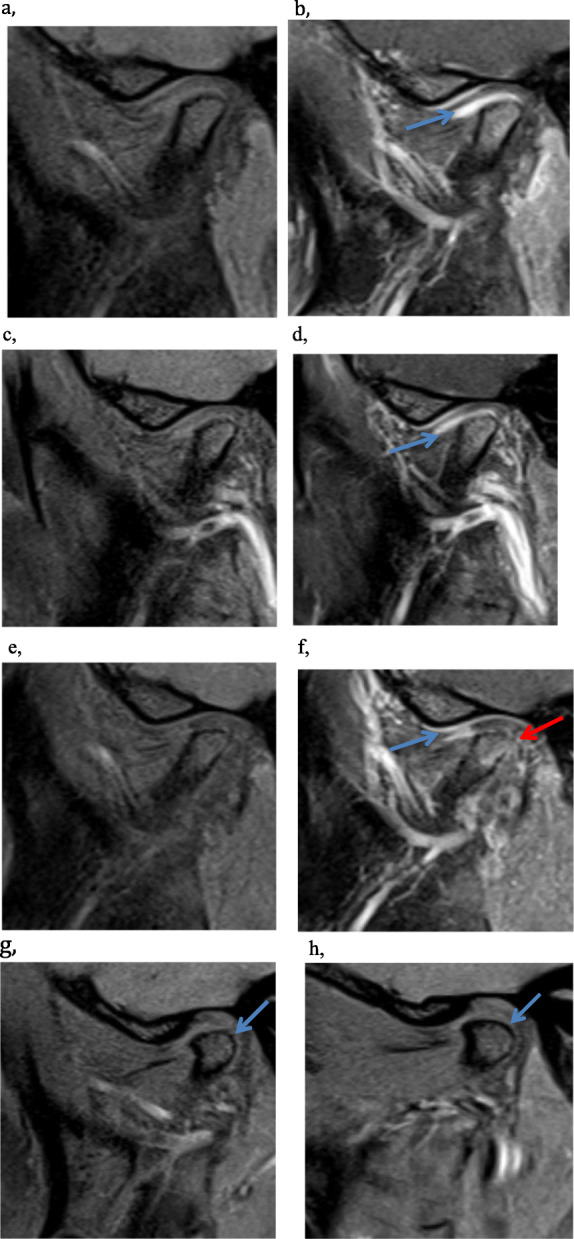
MRI changes of the bone condition. Oblique sagittal pre- and postcontrast T1 TSE images with fat suppression of the left TMJ of a 16-year-old girl (case 10) without improvement in inflammation: joint enhancement is only minimally reduced from baseline (a, b) to 2-months after IAC (c, d). She was under MTX treatment. At 2-year follow up, with a repeated injection 11 months after baseline, there is some reduction in joint enhancement (blue arrow), but the disc has become perforated and the condylar surface discretely more flattened and irregular (red arrow) (e, f). Oblique sagittal T1 TSE images with fat suppression of the right TMJ of a 15-year-old girl (case 9) with improved bone condition: discretely flattened and irregular condyle (blue arrow) at baseline (g) has become smooth and more rounded (blue arrow) at 2-year follow-up after IAC and systemic treatment with biologics and MTX (h)
